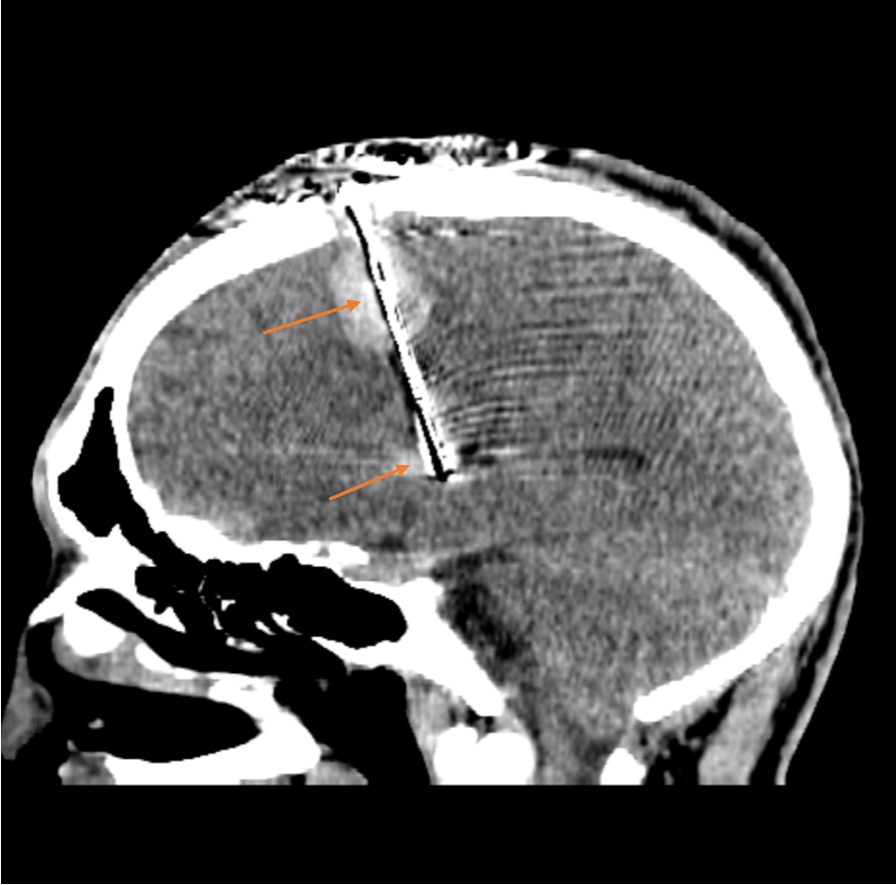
CT scan of brain (fourth postoperative day, sagittal view). Bleeding along the left electrode with maximum in the cortical area, at the site of insertion of the electrode, and in the globus pallidum internum, at the end of the electrode (red arrows). The image quality is partially affected by artifacts from the electrode (electrode – black color; artifact around the electrode - white color)
